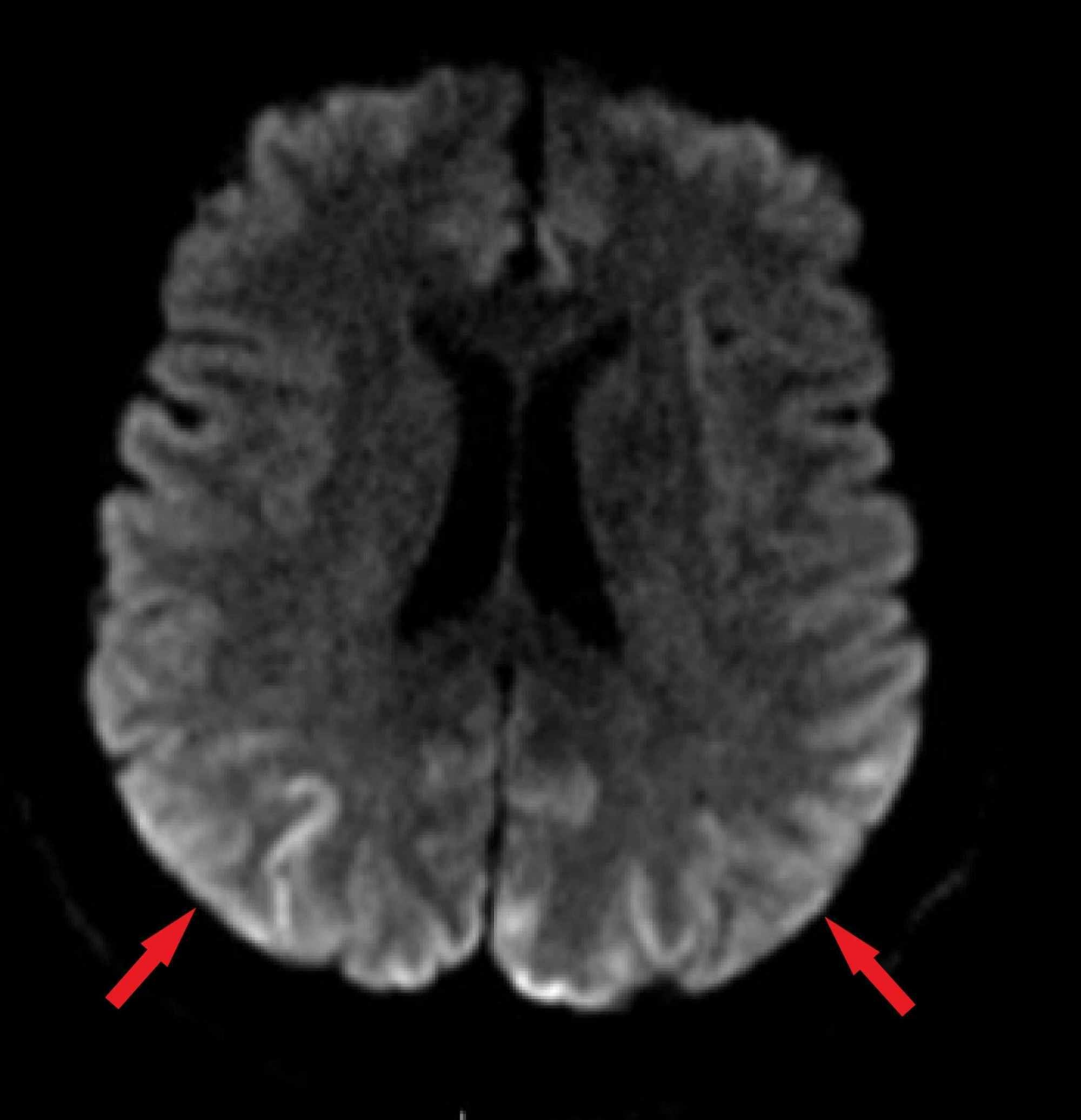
Parieto-occipital Cortical Diffusion Restriction on Axial Magnetic Resonance Imaging
